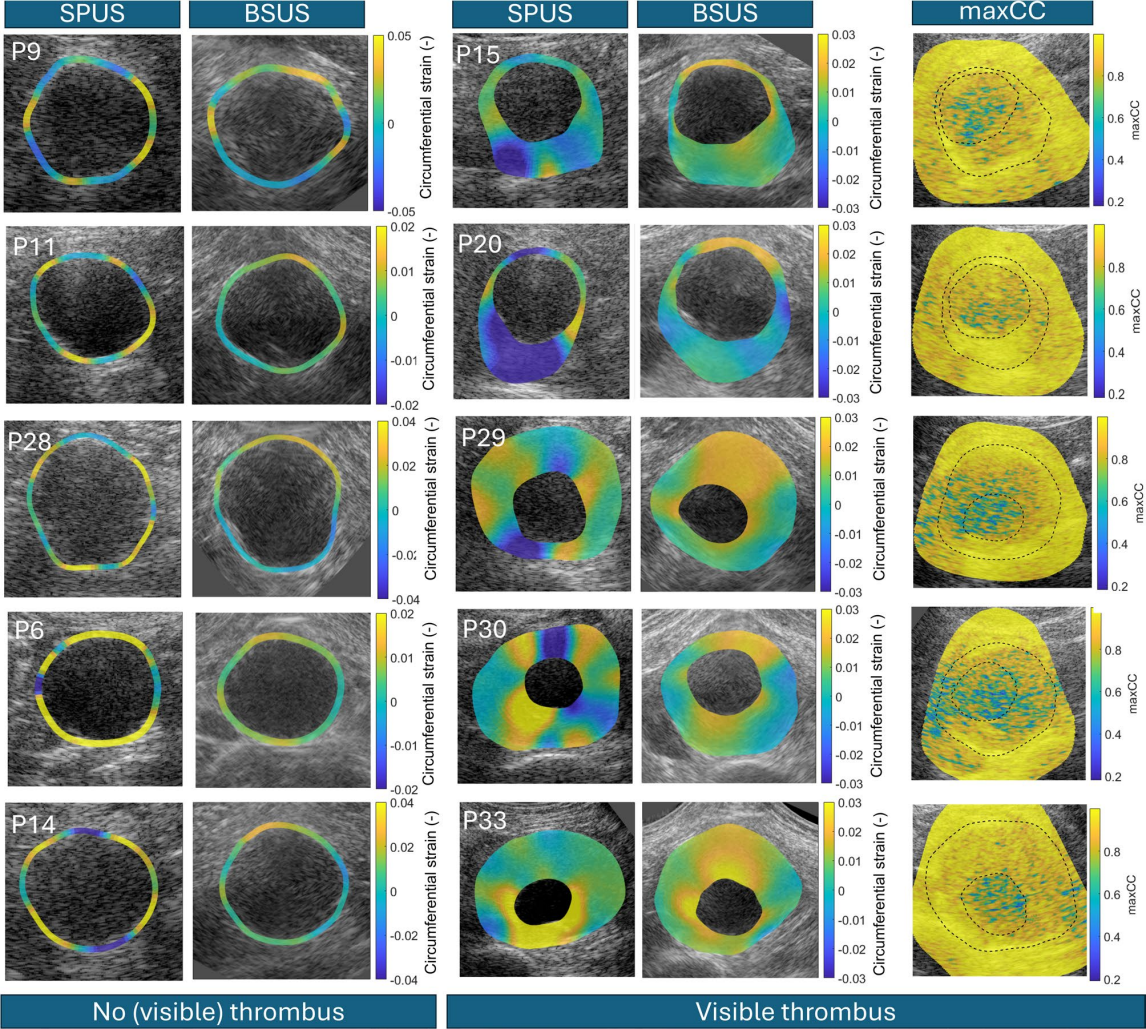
Circumferential strain results at end-systole using single-aperture ultrasound imaging (SPUS) and bistatic dual-aperture ultrasound imaging (BSUS). Left column: results for aneurysms without a visible thrombus. Images are displayed with increasing image quality score from top to bottom. Right column: strain results for aneurysms with visible thrombus. All bistatic US images in this column were scored with fair image quality. Far right: maximum cross-correlation coefficients (maxCC) of the block-matching during systole inside the vessel wall and thrombus in the single-transducer configuration of the bistatic acquisition. The dashed lines indicate the inner and outer border of the segmentation. The images were cropped to fit the aneurysm size: 5$$\times$$5 cm (P9), 6$$\times$$6 cm (P6, P11, P14, P15, P20), 7$$\times$$7 cm (P28, P29, P30), 8$$\times$$8 cm (P33). A video of the measured circumferential strain using BSUS in P15 and P33 is available as supplementary material.
